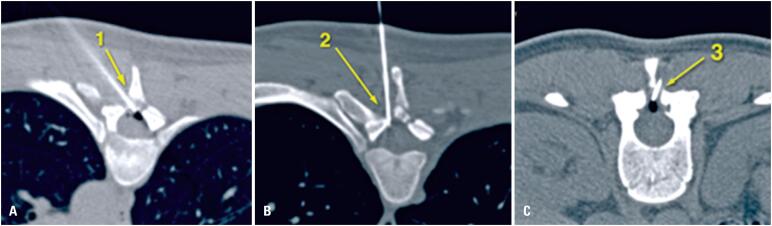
CT-guided interventional epidural blood patches. A) During the first CT-guided epidural blood patch, 6.0mL peripheral blood was injected at the posterior T5-T6 epidural space through a left translaminar approach (1). During the second CT-guided epidural blood patch, B) 15mL peripheral blood was injected at the T3-T4 epidural space (2) and C) 10mL peripheral blood was injected at T12-L1 epidural space (3)
